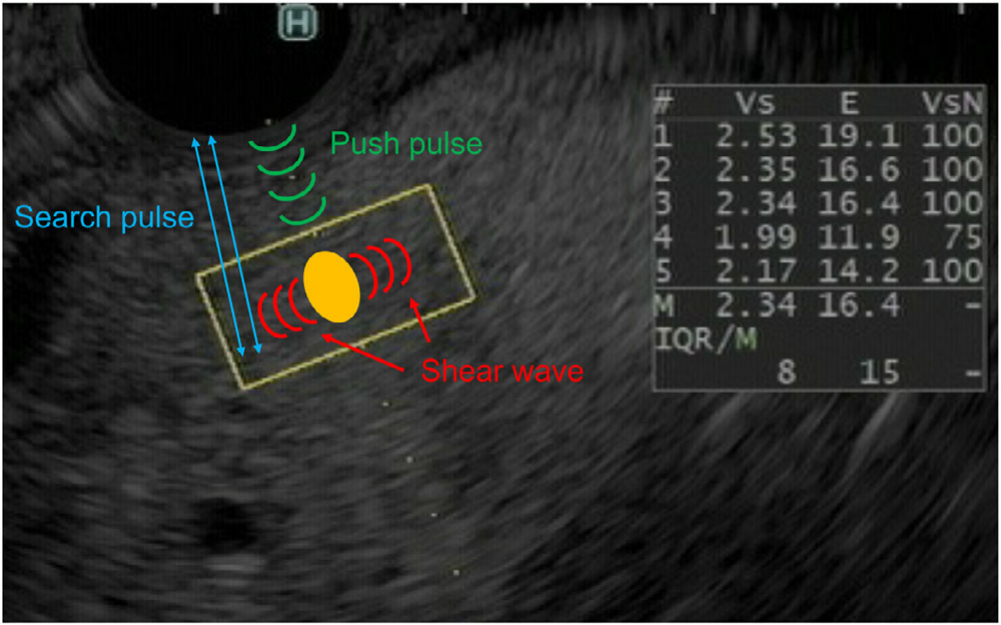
The mechanism of endoscopic ultrasound shear wave elastography (EUS‐SWE). A shear wave is generated by applying a push pulse from the probe to the target organ. The hardness of the organ is estimated by measuring the velocity of the shear wave (Vs, m/s) with a search pulse. The percentage of the net amount of effective shear wave velocity (VsN, %), which indicates the reliability of the Vs, is measured simultaneously.
